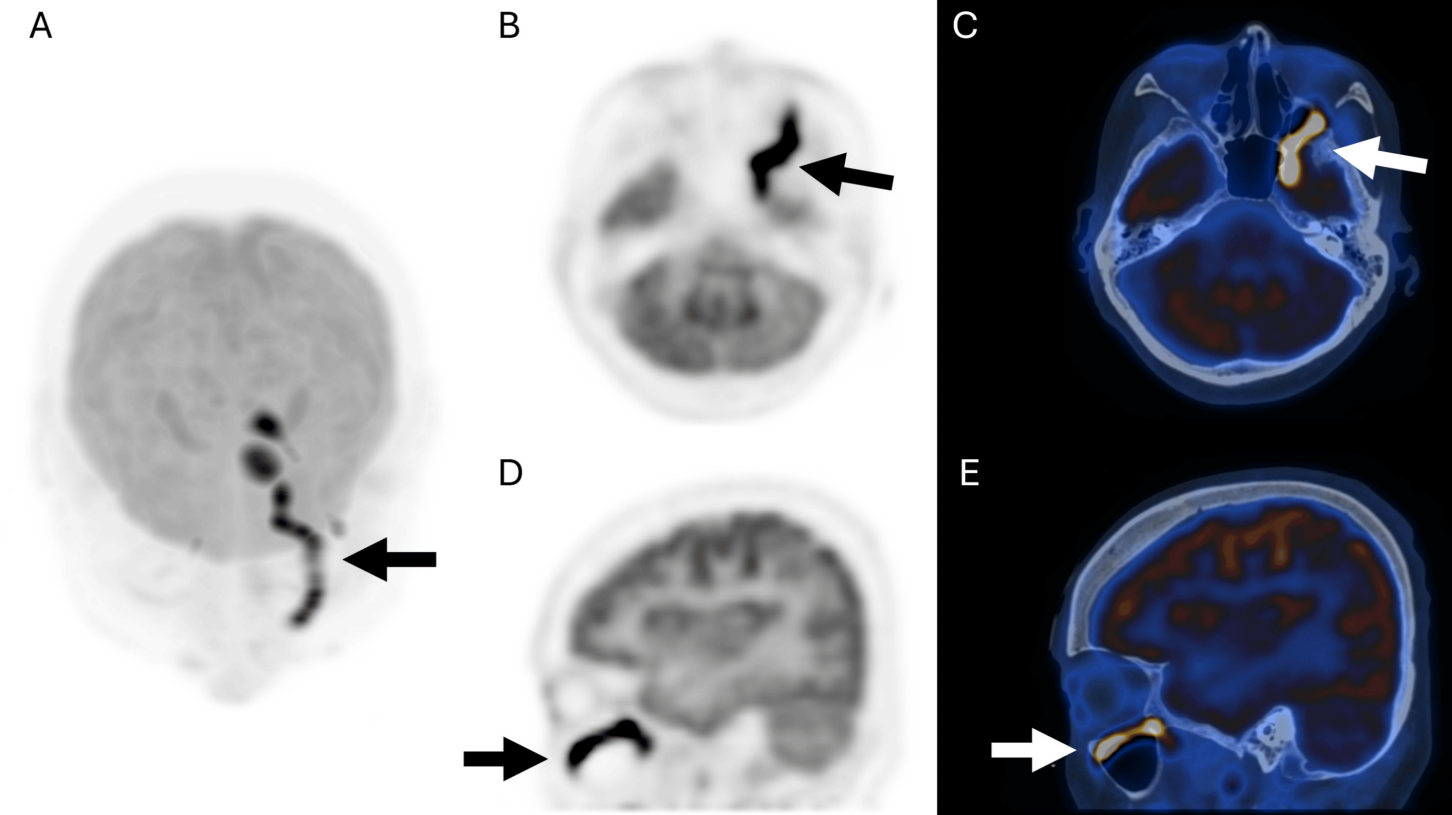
Brain maximum intensity projection image (Top-down view, A) shows multiple hypermetabolic lesions, most strikingly along the maxillary branch (V2) of the left trigeminal nerve. PET-only and PET/CT fusion images showing linear hypermetabolism at left V2 along the inferior orbital fissure (Axial: B-C; Sagittal: D-E).
